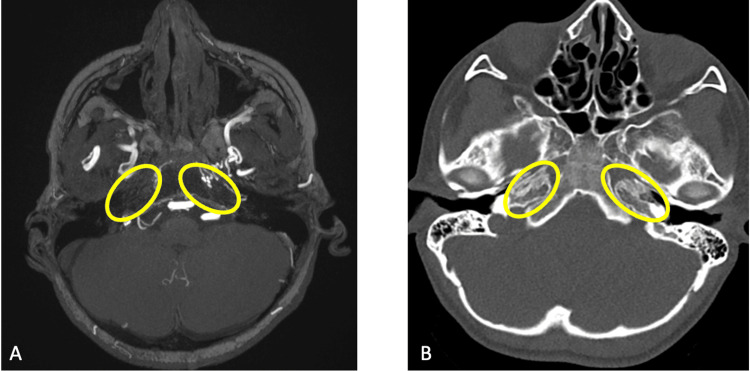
(A) Axial MRA showing absent petrous parts of both internal carotid arteries (yellow circles show ossified canals) and (B) axial CT showing bilateral absent (ossified) carotid canals (yellow circles) MRA: magnetic resonance angiography, CT: computed tomography
